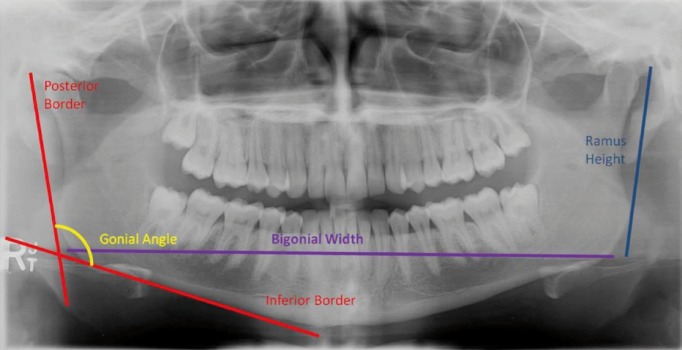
Measurements of the gonial angle, ramus width and bigonial width on panoramic radiographs.
